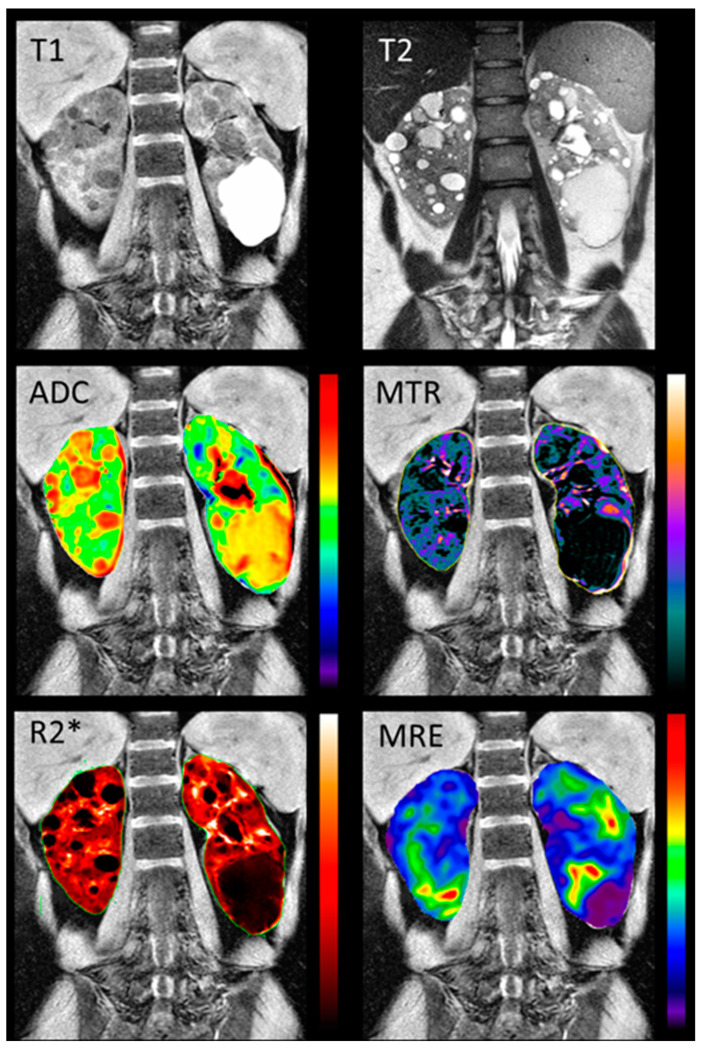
Examples of the multiparametric MRI of the kidney in a representative ADPKD patient. The top row shows structural MRI scans (T1- and T2-weighted). The second and third rows show quantitative maps, overlaid on the T1-weighted scan: the apparent diffusion coefficient ‘ADC’ derived from DWI, the magnetization transfer ratio ‘MTR’ derived from MTI, the apparent transverse relaxation rate ‘R2*’ derived from BOLD, and the tissue stiffness map derived from MRE. The figure was reprinted with permission from Kline, T.L. et al. Quantitative MRI of kidneys in renal disease [87].
